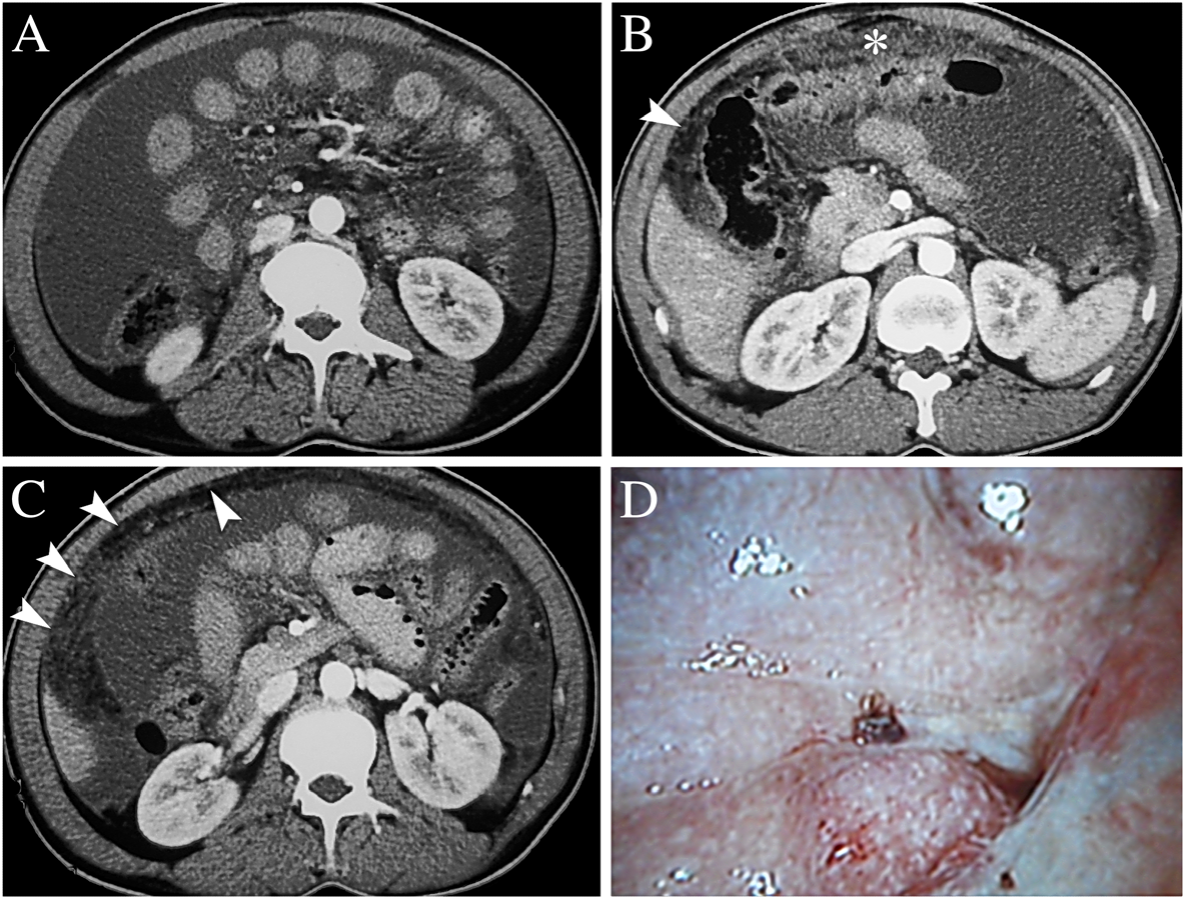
a: CT scan showed the presence of well-encapsulated fluid collection and central accumulation of the small intestine; b, c: CT scan revealed smudged appearance of the greater omentum (*), as well as multiple small nodules and sheetlike lesions on the parietal peritoneum (arrowhead); d: Laparoscopy demonstrated that the entire small bowel was encapsulated in a dense, white, fibrous, cocoon-like membrane associated with a large amount of brownish ascites. Multitudinous miliary nodules or ubercles were seen on the parietal peritoneum
